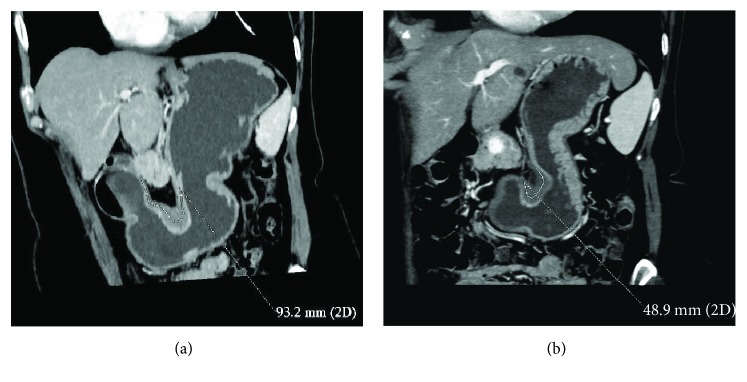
(a, b) GC cancer of the angulus (diffuse histotype) in a 69-year-old woman. TRG 2 was found on final pathology after NAC. Tumour D-max reduction rate (47.5%), between CT before (a) and after NAC (b), correctly identified TRG.
